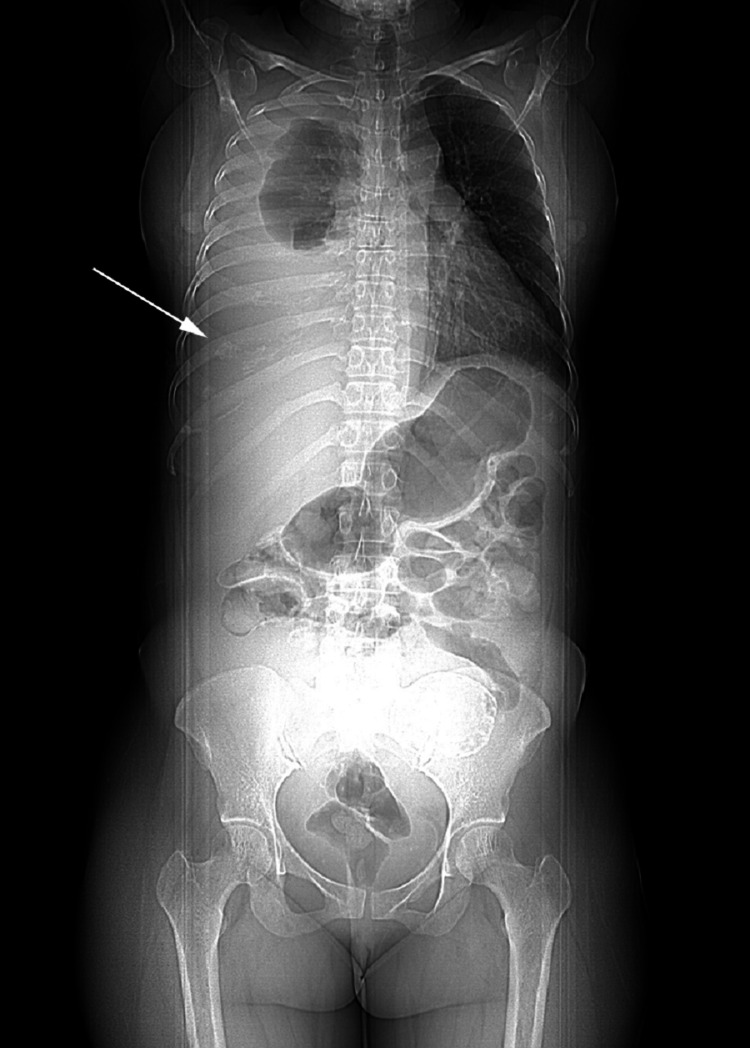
Chest X-ray taken in the supine position showing the effusion
